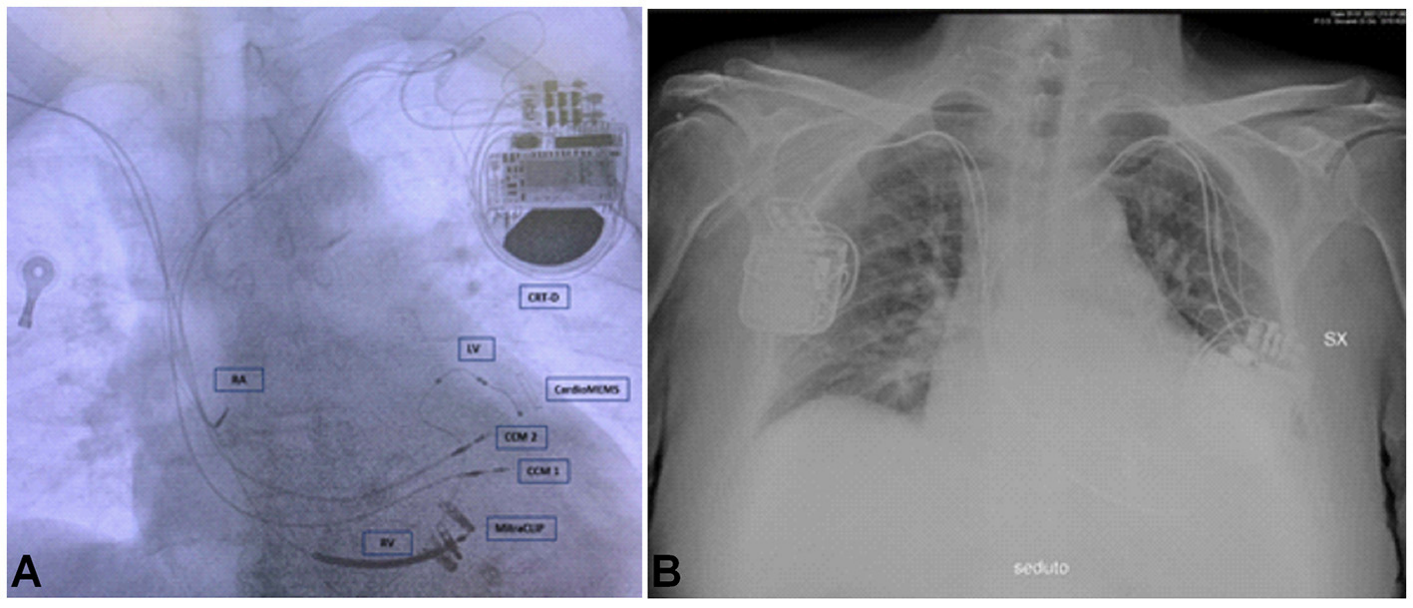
Chest X-ray of the patient. (A) The figure shows two CCM leads, three CRT-D leads, CardioMEMS, and MitraClips. (B) The figure shows CCM device on the right and CRT-D on the left. CRT-D, cardiac resynchronization therapy – defibrillator device; CCM 1, lead 1 CCM; CCM 2, lead 2 CCM; RV, right ventricular lead for CRT-D; LV, left ventricular lead for CRT-D; RA, right atrial lead for CRT-D.
